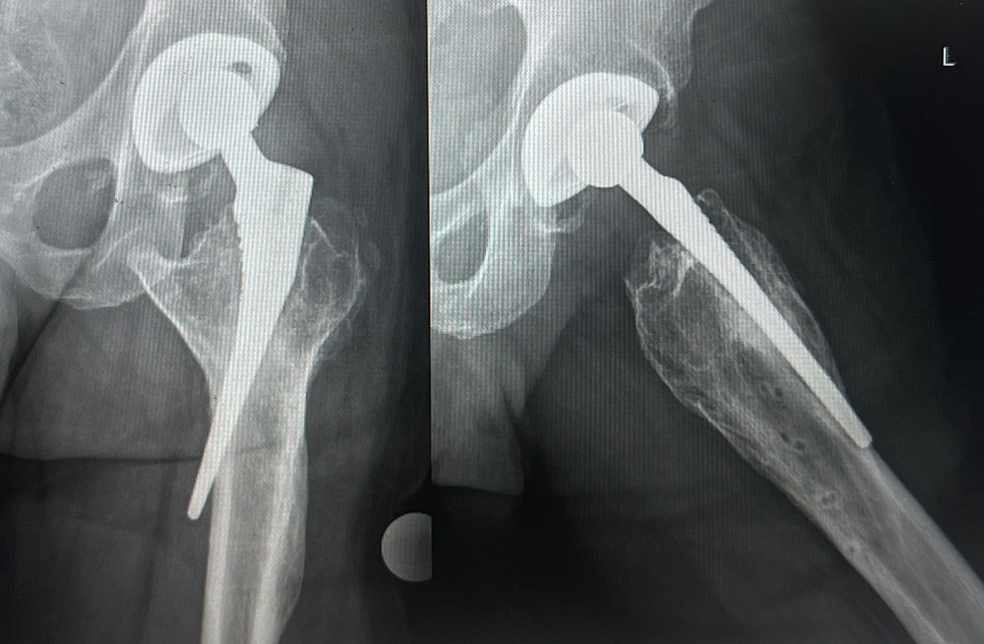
Anteroposterior and lateral hip radiographic images demonstrating femoral component malpositioning and perforation
